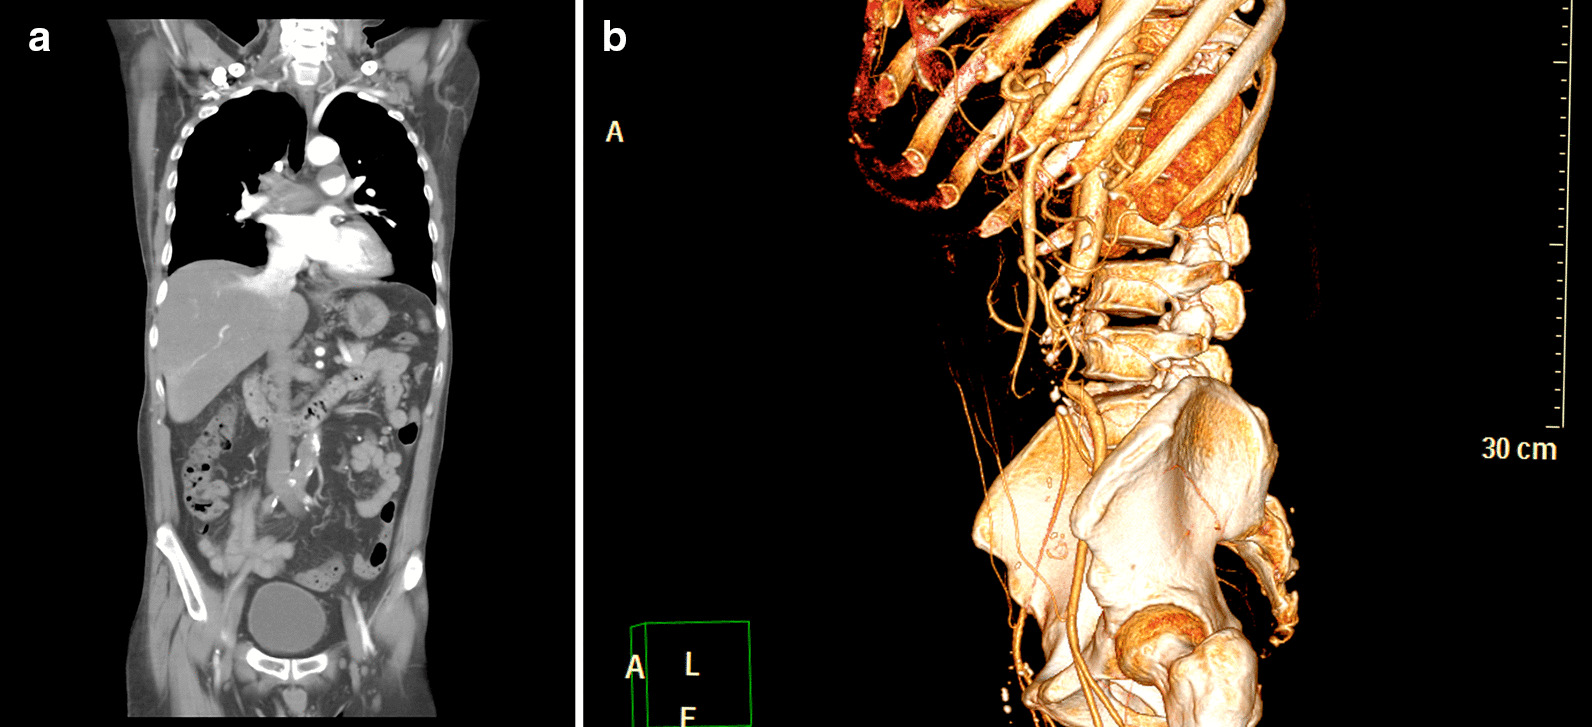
Computed tomography angiography shows a a massive thrombus straddling both the pulmonary arteries, with aortoiliac occlusion disease. b However, there is no obvious establishment of collateral vessels around the occluded vessels from the infrarenal abdominal aorta to the bilateral common iliac arteries
